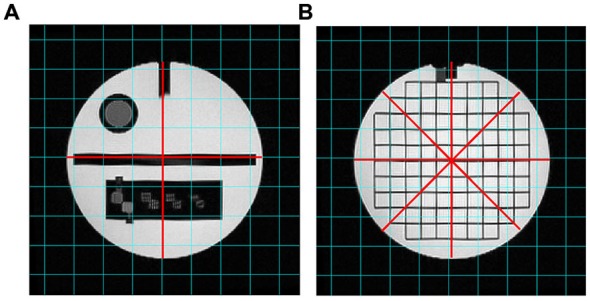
T2-weighted images analyzed for the “Geometric accuracy” test. The red lines indicate the diameters to be measured on slice 5 (A) and slice 12 (B).
